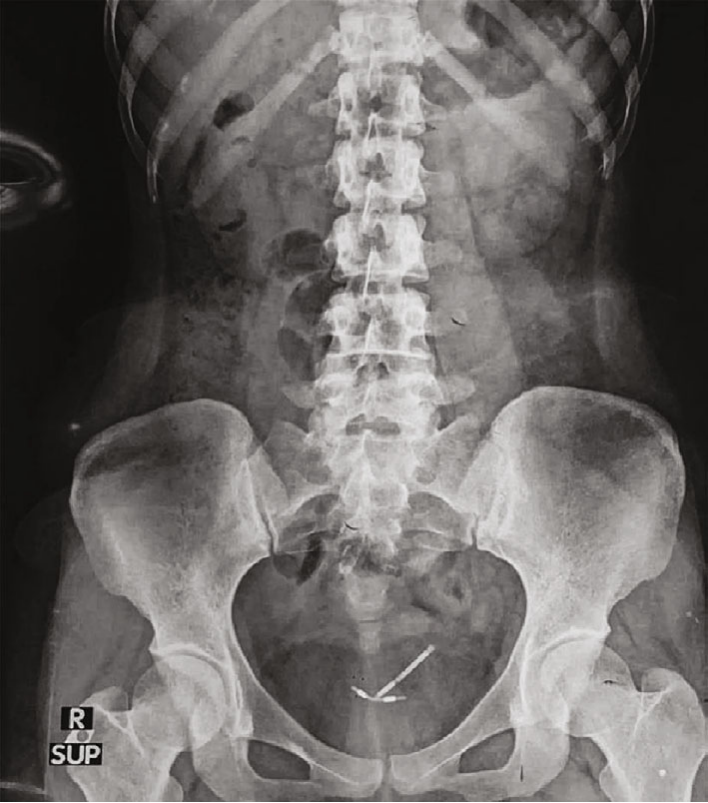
The abdominal plain X-ray revealed that the IUCD was visualized within the pelvic cavity (white arrow).
